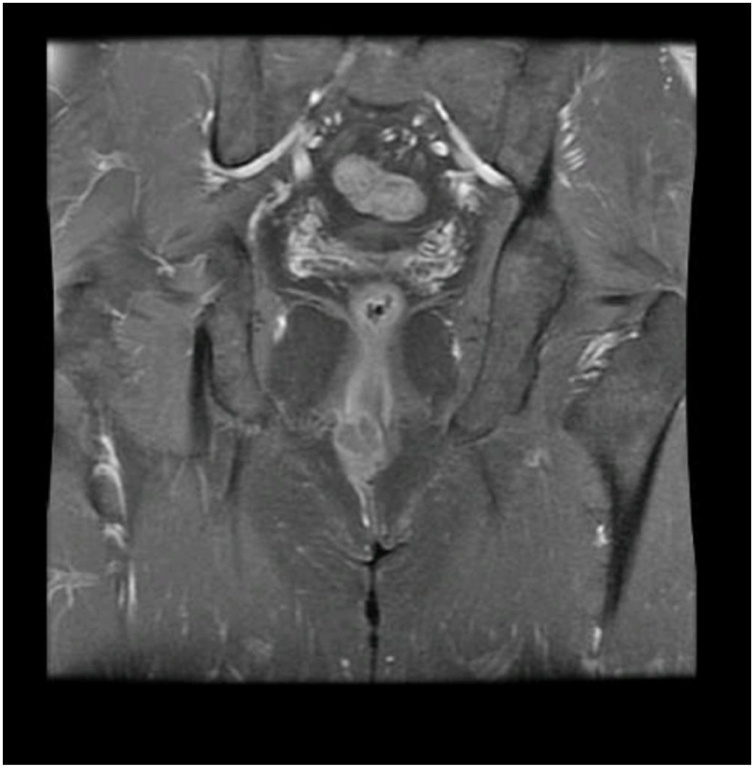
Coronal plane of pelvic MRI showing the mass.
